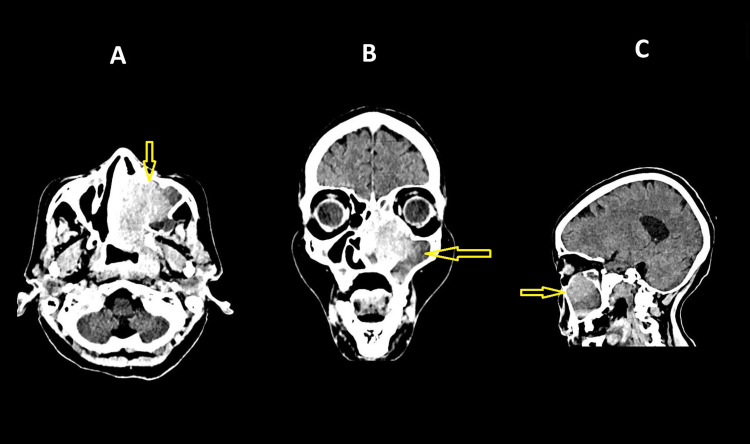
Contrast-enhanced CT of the paranasal sinuses Axial (A), coronal (B), and sagittal (C) contrast-enhanced CT images show a heterogeneous soft tissue mass (yellow arrows) occupying the left maxillary sinus, with destruction of the palatal bone and extension toward the pterygoid plate region, consistent with an aggressive sinonasal neoplasm. Based on imaging, the lesion corresponds to clinical stage cT4aN2cM0.
